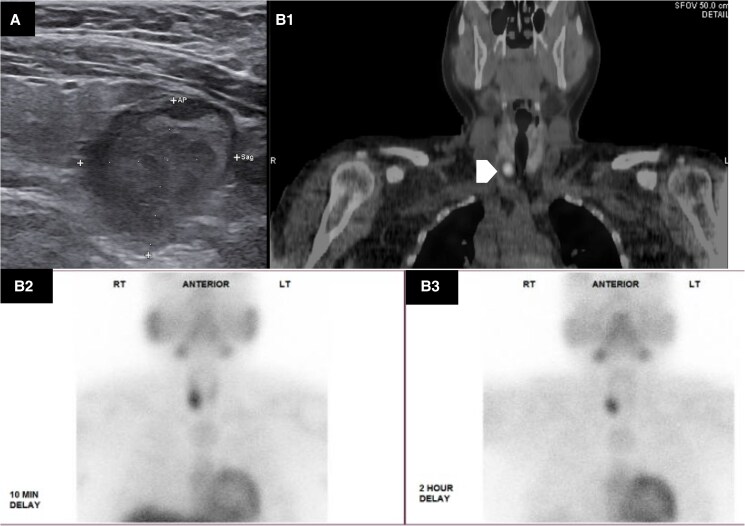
Preoperative imaging assessment. Preoperative ultrasound (A) showed a 1.95 × 1.25 × 5.31 cm right inferior parathyroid lesion. This lesion was also identified by sestamibi scan with 4-dimensional computed tomography (B1, white arrowhead) and showed increased radiotracer activity on 2-hour delayed planar imaging (B2-B3, where B2 represents 10-minute delay and B3 represents 2-hour delay).
